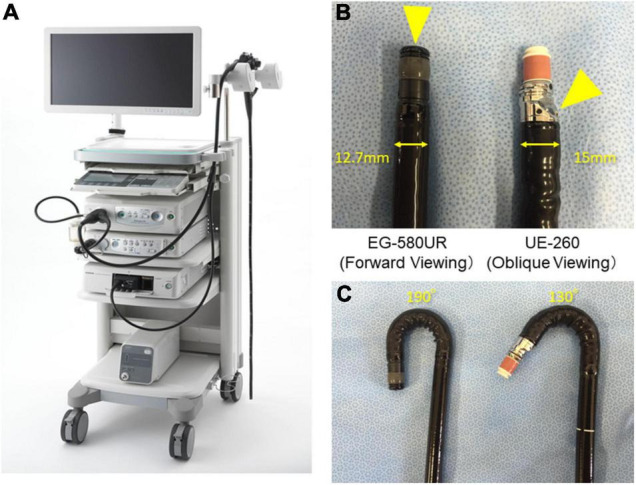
Endoscopic ultrasound (EUS) system and differences between forward-viewing radial EUS (FR-EUS) and conventional radial EUS. (A) EG-580UR with SU-1 EUS System (Fuji Medical, Tokyo, Japan). (B) The optical lens is located at the front in FR-EUS but behind the ultrasound transducer in conventional radial EUS. (C) The degree of up angle of FR-EUS is 190° but 130° in conventional radial EUS.
